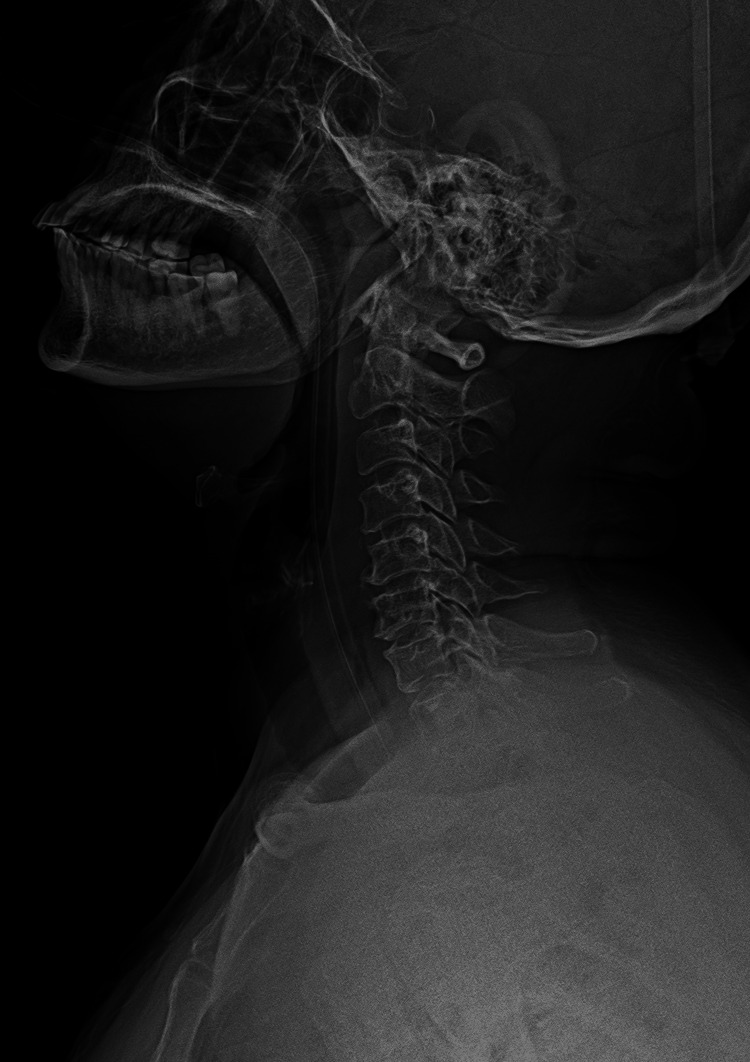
Post-op X-ray neck lateral view (soft tissue), Ryles tube in-situ.
